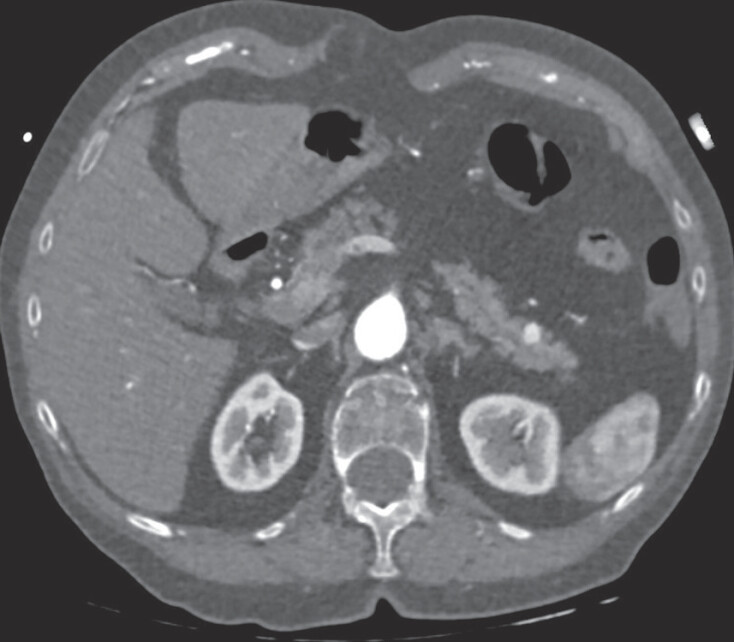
The CT scan appearance of the solid pancreatic lesion.
